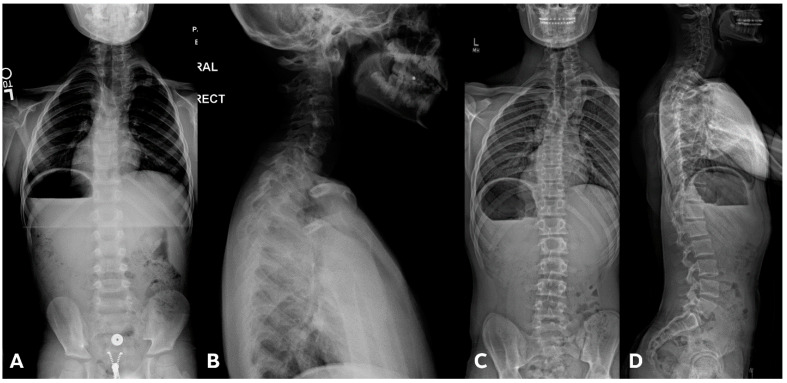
(A,B) Initial PA and lateral radiograph of a 7-year-old child with a 19° cervicothoracic scoliosis and T1 slope of 31°. (C,D) Follow-up PA and lateral radiographs showing progression of the curve to 38° over 8 years.
